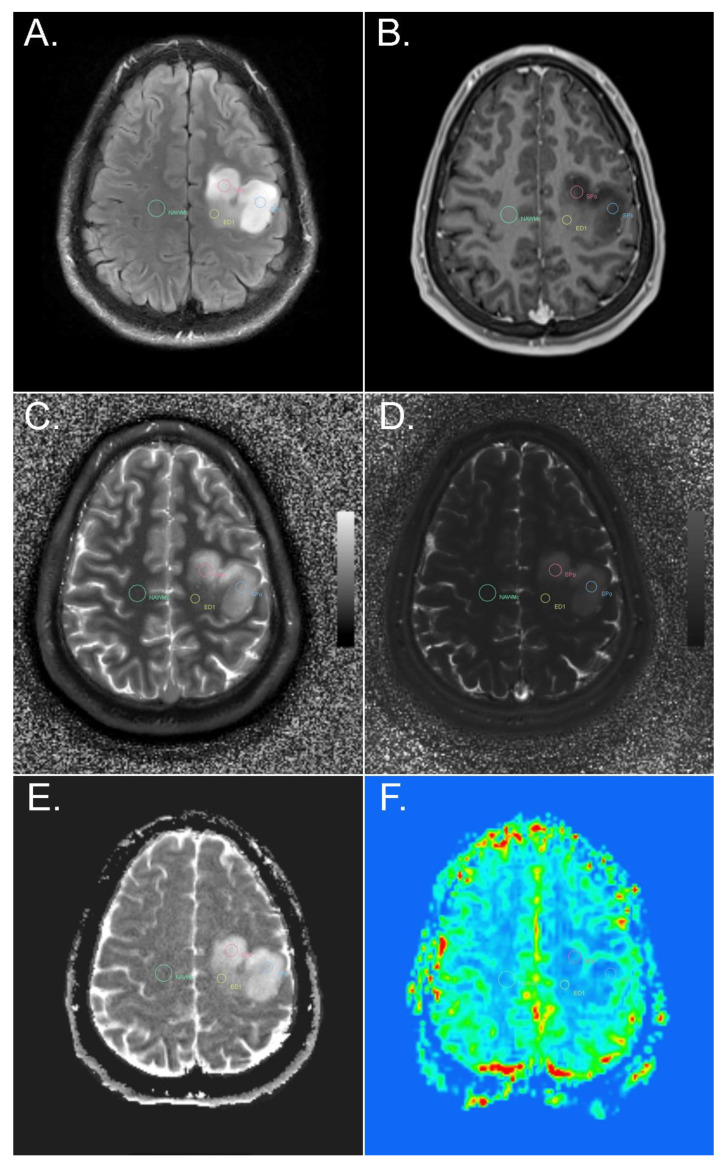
Example of ROI placement in different tumor and peritumoral components of a diffuse astrocytoma, IDH-mutant, in a left precentral location. FLAIR (A), T1-weighted MPR post i.v. contrast administration (B), MRF T1 (C) and T2 (D) maps, ADC map (E), and perfusion-weighted imaging (F). ROIs were placed in the solid tumor part without contrast enhancement and without an increase in rCBV (red and blue circle), in peritumoral edema (yellow circle), and in contralateral NAWM (green circle). SPo, solid part of the tumor without contrast enhancement; ED1, peritumoral edema less than or equal to 1 cm distant from the tumor; NAWMc, contralateral NAWM of the frontal lobe.
